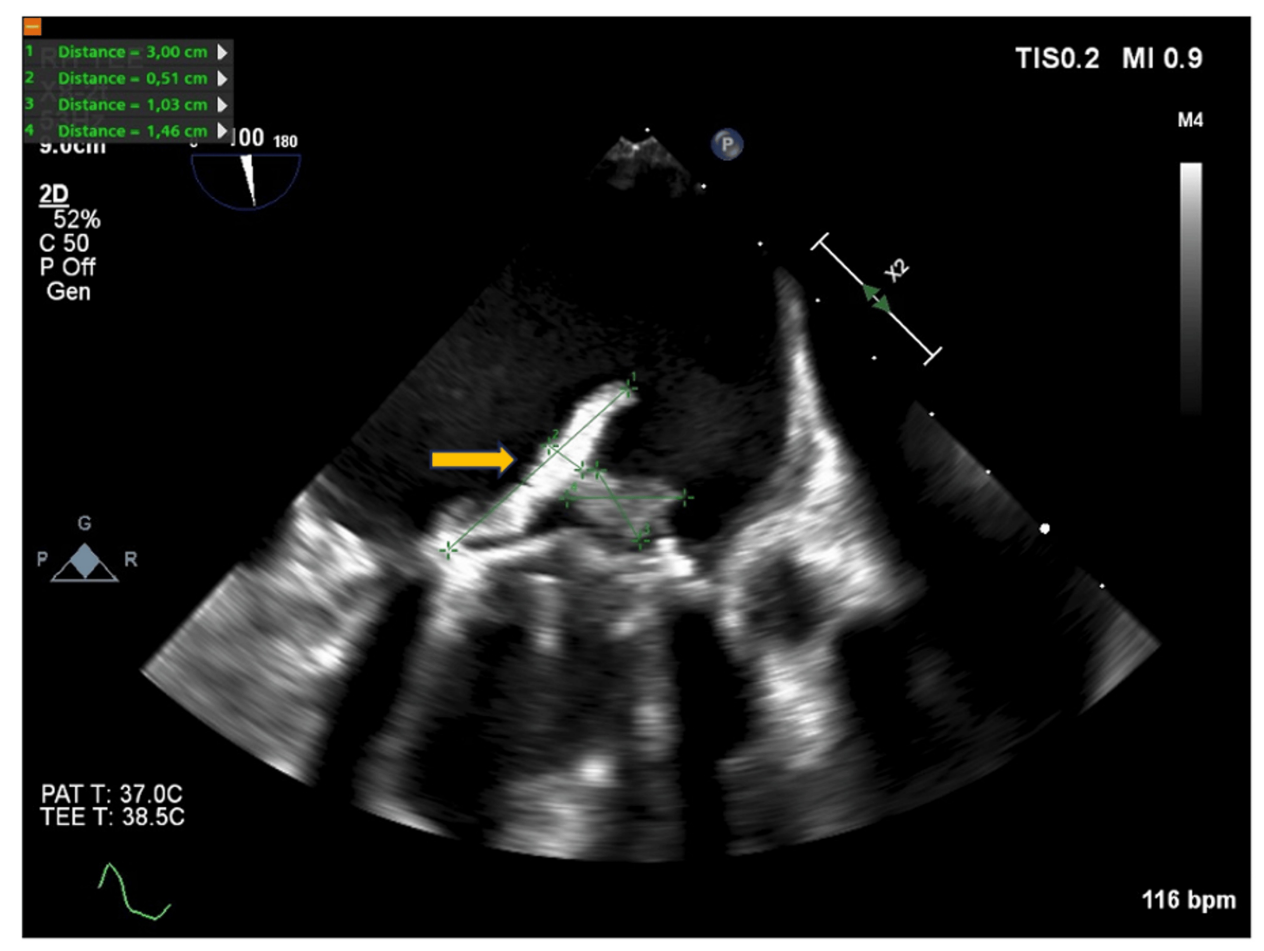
A transesophageal echocardiography (TEE) of the prosthetic mitral valve with visualization of the progression 23 day after hospital admission and prior to surgery Arrow shows vegetation with measurements
